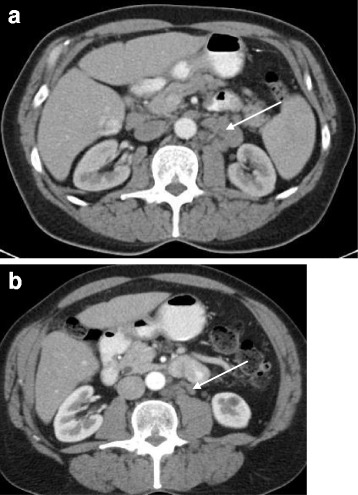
68-year-old male patient with relapsed CLL on Obinitizumab. a Axial CT images of the abdomen obtained in portal venous phase demonstrates prominent left para aortic lymph node measuring 3.4 × 1.6 cm (white arrow). b Axial CT images of the abdomen obtained 6 months after therapy demonstrate complete response with the lymph node measuring 1.7 × 0.9 cm (white arrow)
